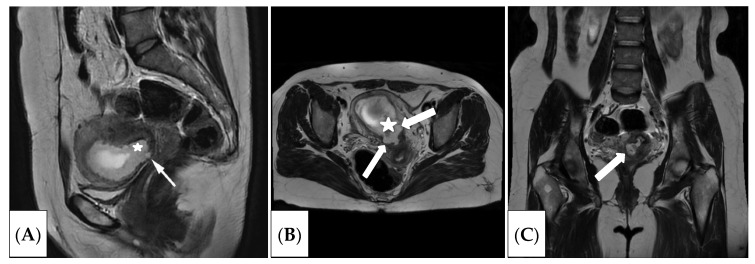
MRI T2WIs in the sagittal (A), axial (B), and coronal (C) planes showing the gestational sac within the uterine cavity. The chorion was identified in the isthmus of the uterus (indicated by an asterisk), with fragments extending into the scar from the caesarean section and the isthmus of the uterus (indicated by a white arrow).
